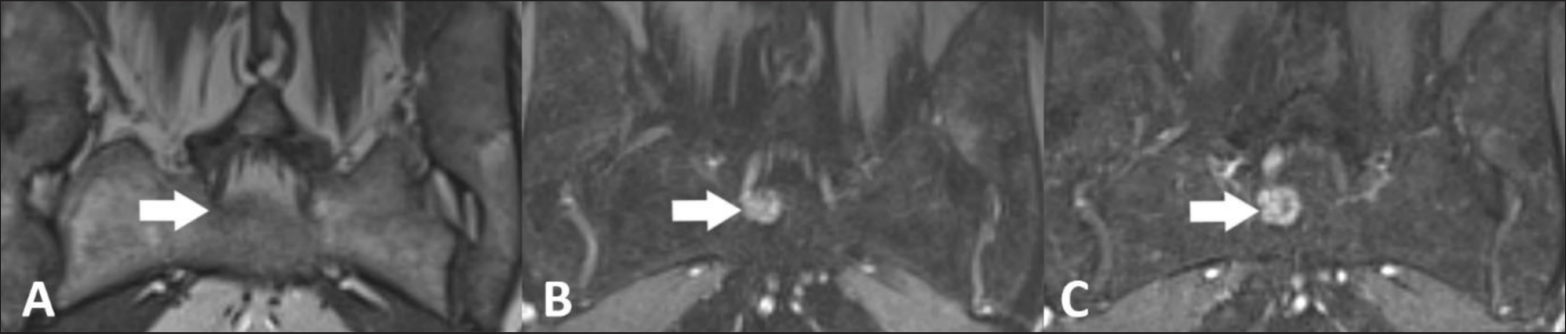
A 51-year-old male with small sacral enchondroma (arrow). (a) Coronal T1-weighted MR image shows a hypointense mass in the body of the sacrum. (b) Coronal fat-saturated T2-weighted MR image shows a lobulated hyperintense mass. Note absence of bone marrow oedema. (c) Coronal fat-saturated post-contrast T1-weighted MR image shows septal enhancement.
